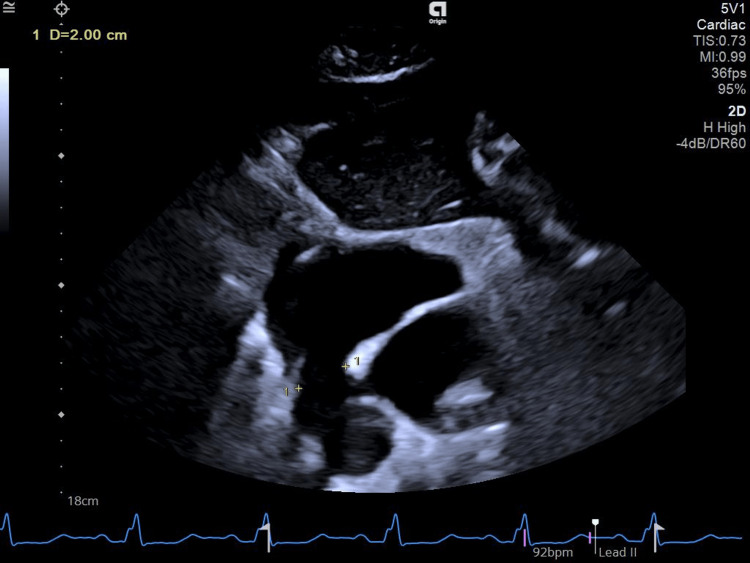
Transesophageal echo showing an atrial septal defect (ASD) of 2 cm
